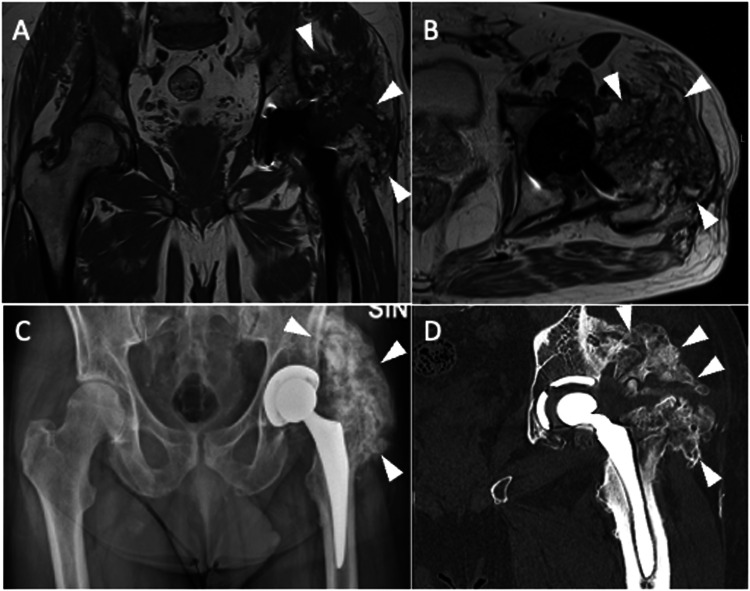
Heterotopic ossification of let THA in a 72-year-old male patient. Coronal T1 (A), axial T2-weighted (B), AP X-ray view (C), and coronal CT (D) images show periprosthetic heterogeneous mass with an ossified component which appears hyperintense on both T1- and T2-weighted images (arrowheads). Further, bone resorption is observed along the femoral stem in CT image (D)
